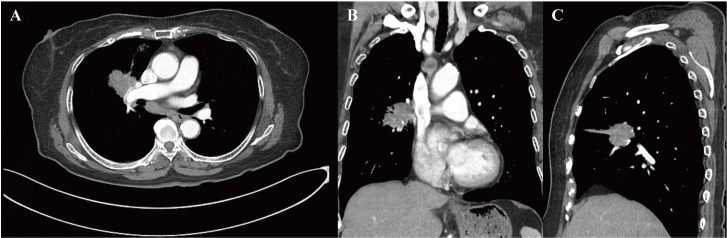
Baseline computed tomography (CT). (A–C) Baseline axial, coronal, and sagittal CT views revealing a 3.5-cm central mass in the right upper lobe.
